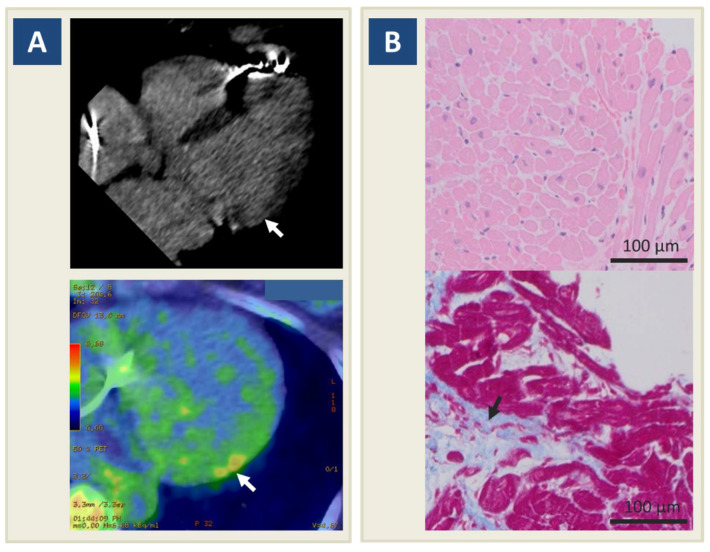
Follow-up restaging of myocarditis. Legend. (A) Cardiac computed tomography shows subepicardial late iodine enhancement in the lateral left ventricular wall (upper panel, arrow), corresponding to baseline findings on cardiac magnetic resonance; at the same time, 18-F fluorodeoxyglucose positron emission tomography scan shows no remarkable myocardial inflammatory activity at the same site (lower panel, arrow). (B) Follow-up endomyocardial biopsy shows rare lymphocytic inflammatory cells (CD3+ < 7 mm^2^), lack of edema and no cardiac myocyte necrosis (upper panel); fibrosis is the dominant component (lower panel, arrow).
